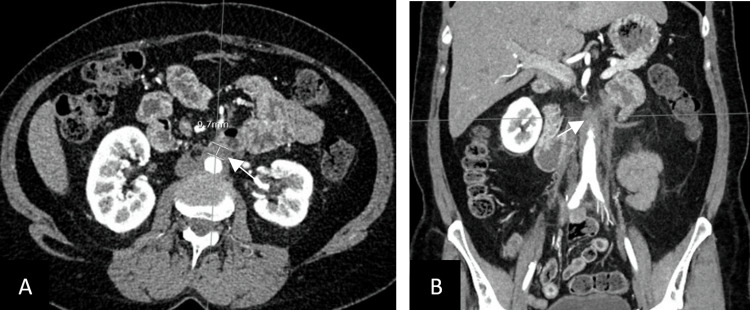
Axial (A) and coronal (B) slices of CT angiogram depicting thickening and infiltration of the abdominal aorta (white arrows) with periaortic fat infiltration.
